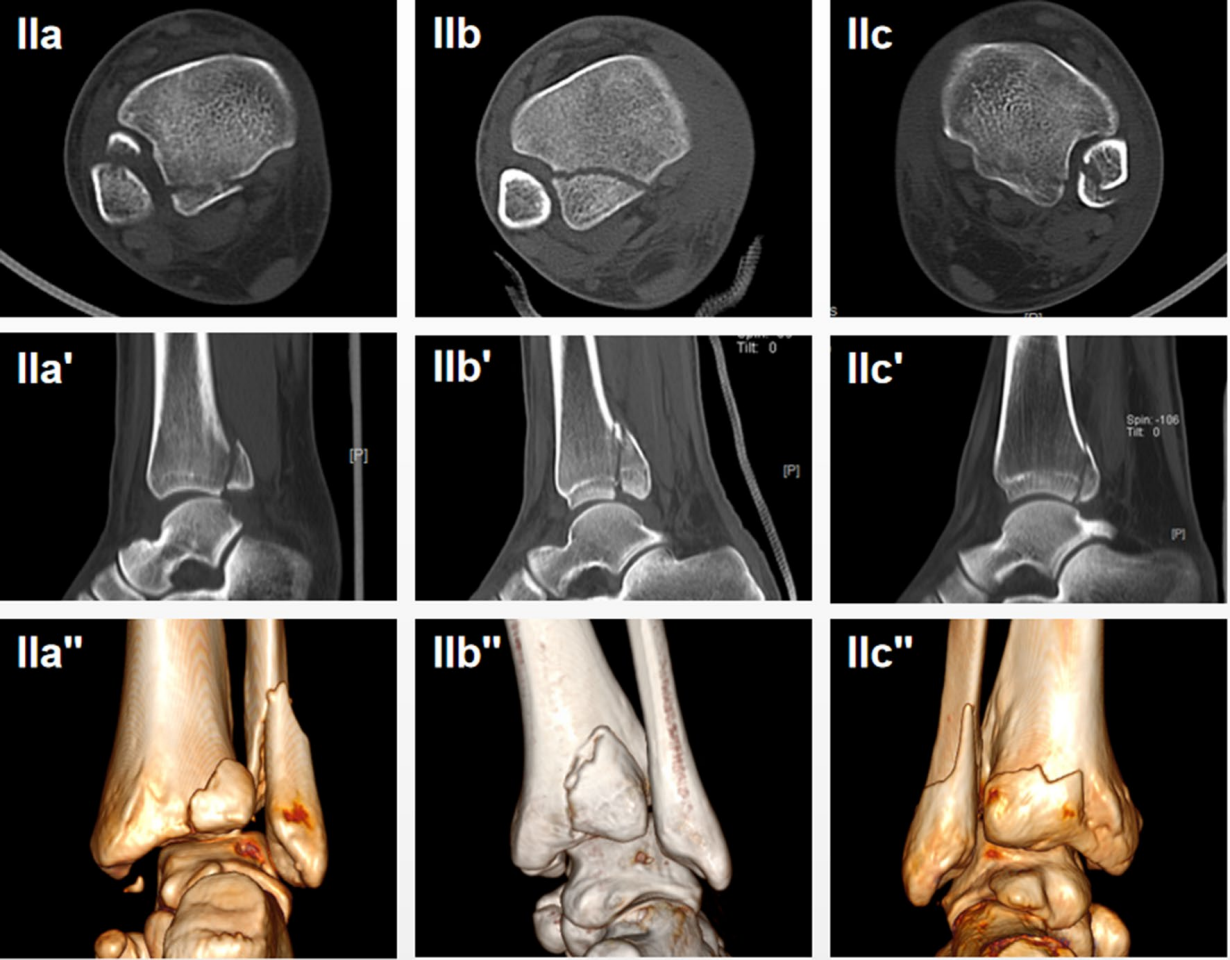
Variability of Bartonicek classification type II PMF (intraincisural posterolateral fragment) on CT scans in horizontal plane (IIa, IIb, IIc), sagittal plane (IIa′, IIb′, IIc′) and 3D reconstruction (IIa″, IIb″, IIc″)
